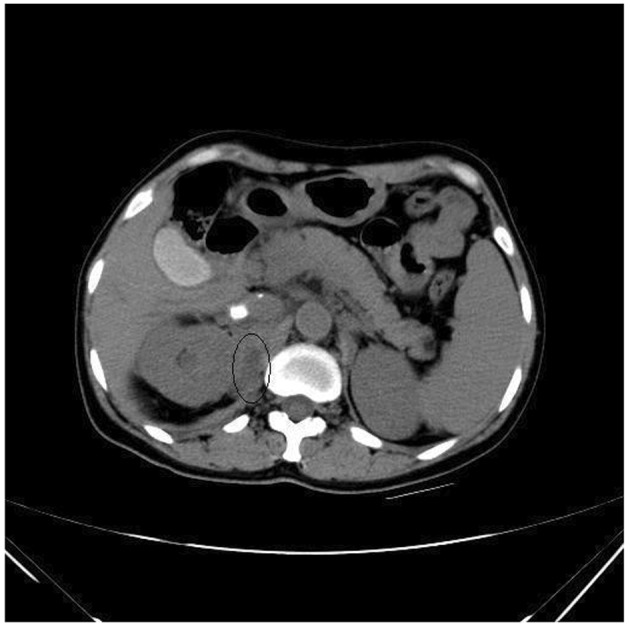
Computed tomography scan revealing a mass in the retroperitoneal region.
